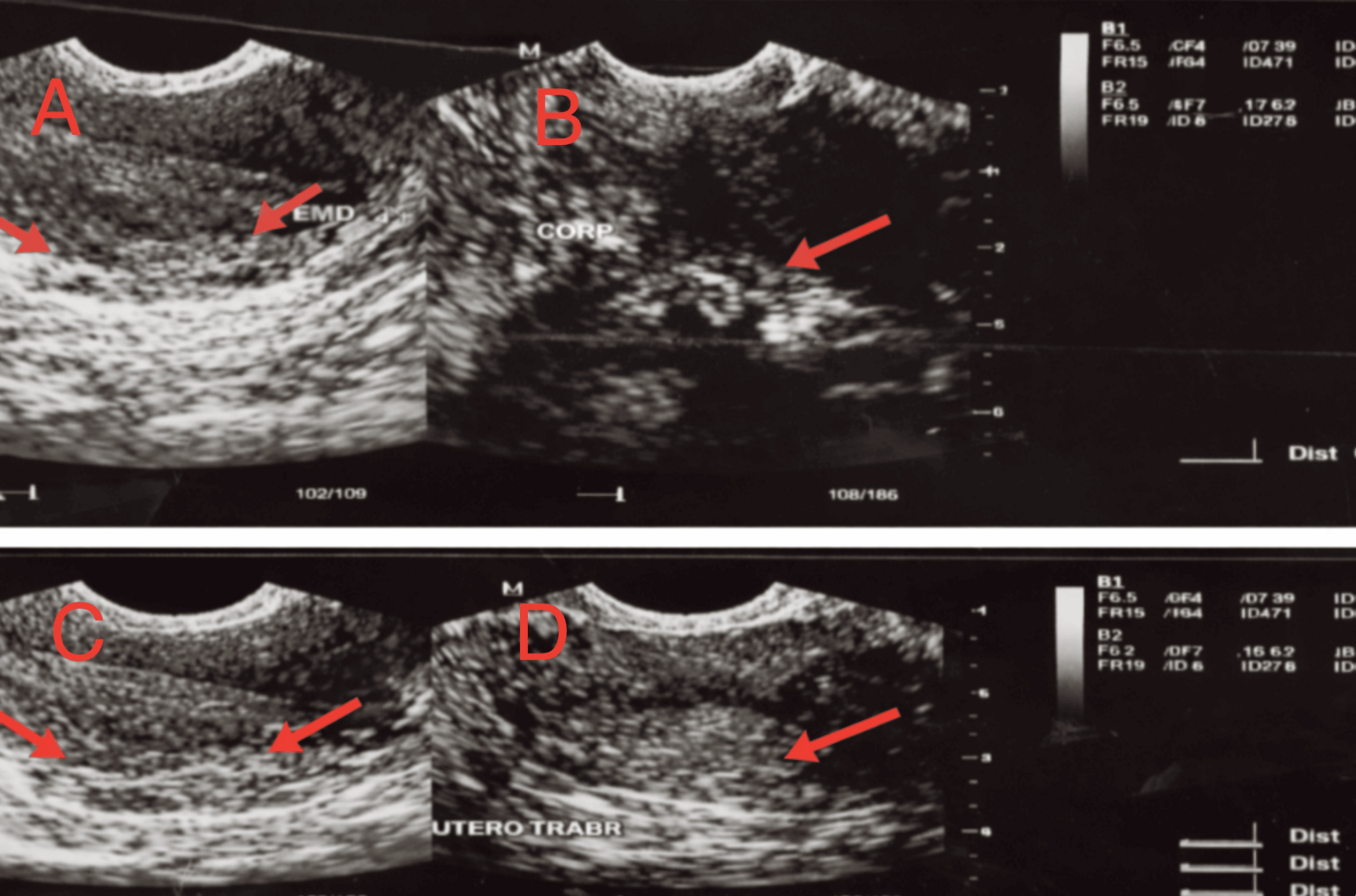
Transvaginal ultrasound evaluation of the uterus and endometrium (left to right) (A) Longitudinal view of the uterus demonstrating the endometrial stripe with measured thickness of approximately 0.76 cm.
